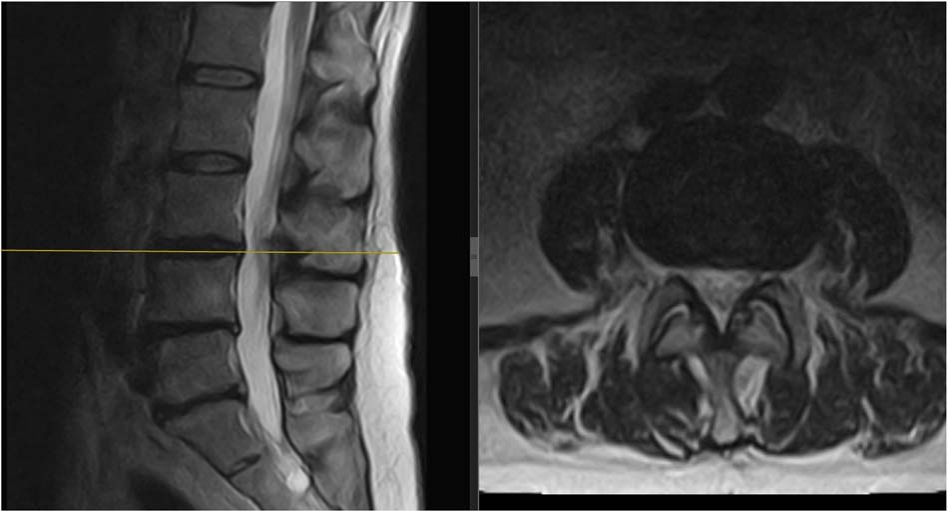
Follow-up MRI scan of the lumbar spine: follow-up MRI scan of the lumbar spine performed in March 2023 showing complete resolution of the disc prolapse.
